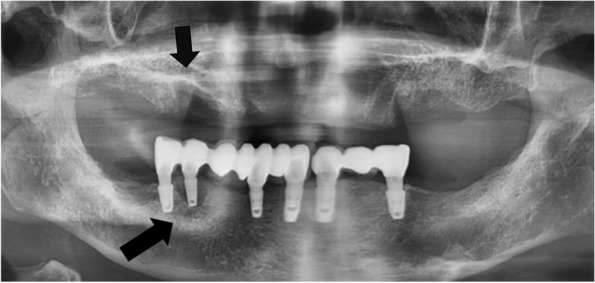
Panoramic radiograph. Osteomyelitis around the socket of the right anterior maxilla after tooth extraction, as well as a radiolucent lesion below the #44 implant
